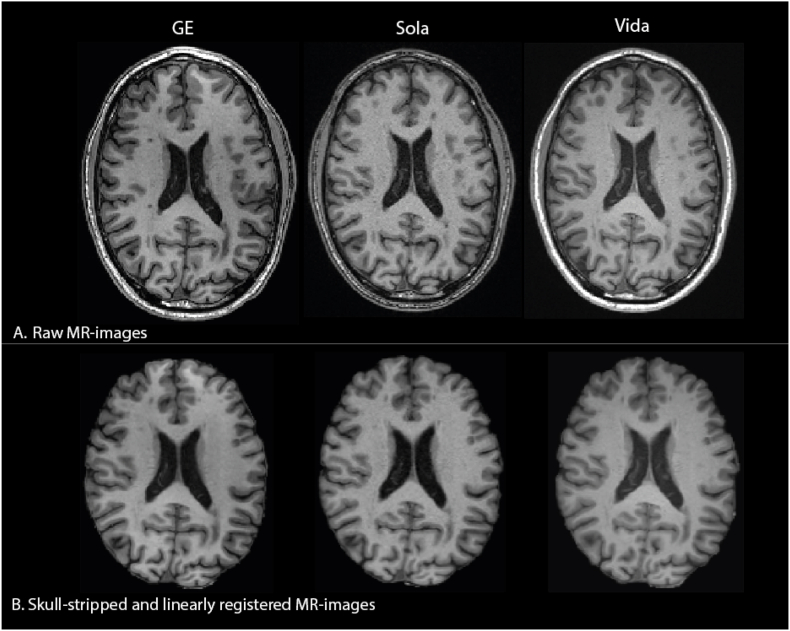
A*Example of the raw T1w MR images of a pwMS (male, age* = *57.8 years, disease duration* = *2.9 years, EDSS* = *2, phenotype* = *RRMS).BPre-processed derivatives ofAafter skull-stripping (HD-BET) and linear registration to standard MNI space (FSL-FLIRT).*
